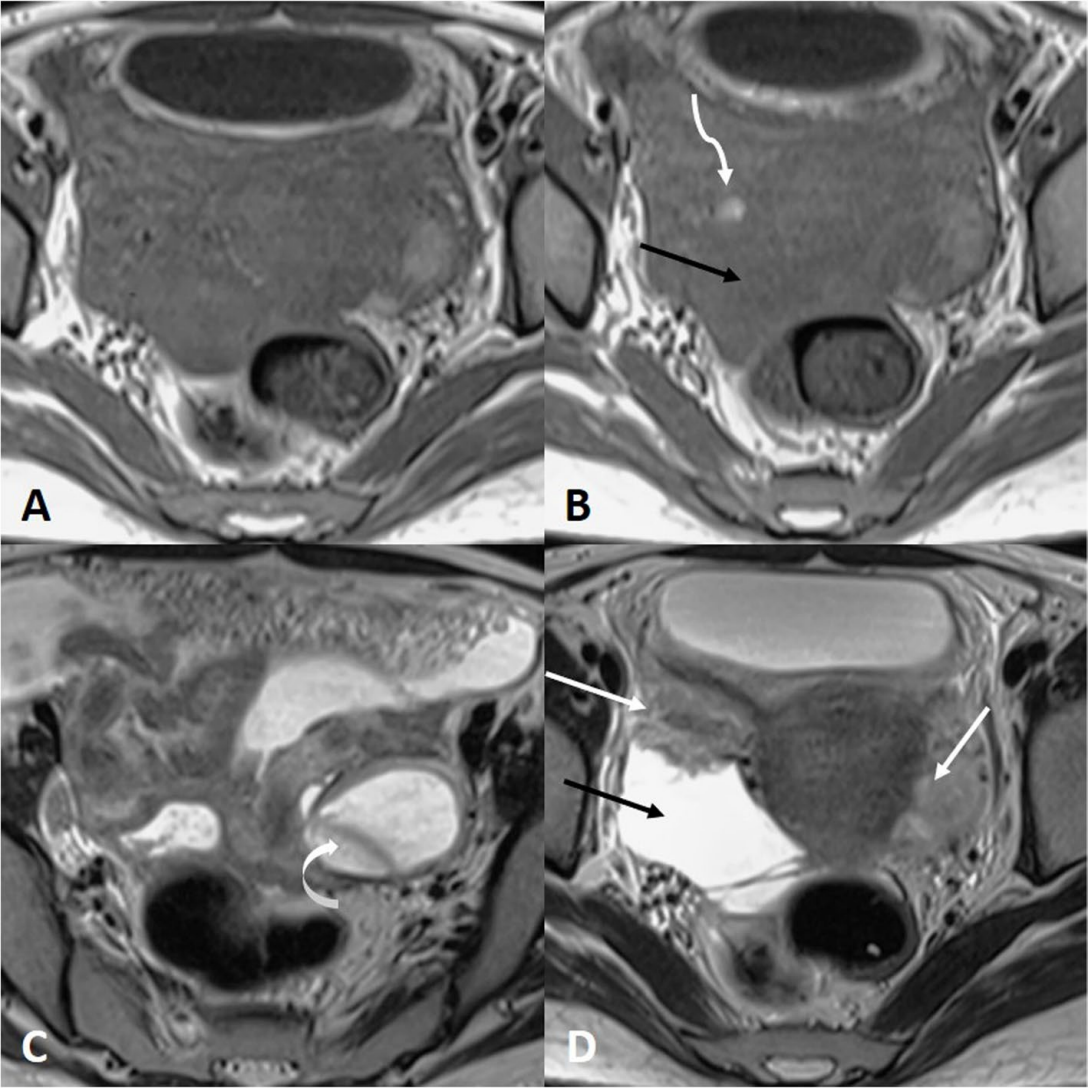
Axial MRI images of the pelvis after treatment showing the uterus in the center with near-total resolution of previously seen mass. Only a small component which has verted to the left side (curved arrow) is remaining. The previously seen calcification is still noted (twisted arrow). The bulk of both ovaries is however visualized normally (straight white arrow). Mild ascites is seen (straight black arrow) which may indicate post-treatment residual peritumoral inflammation
